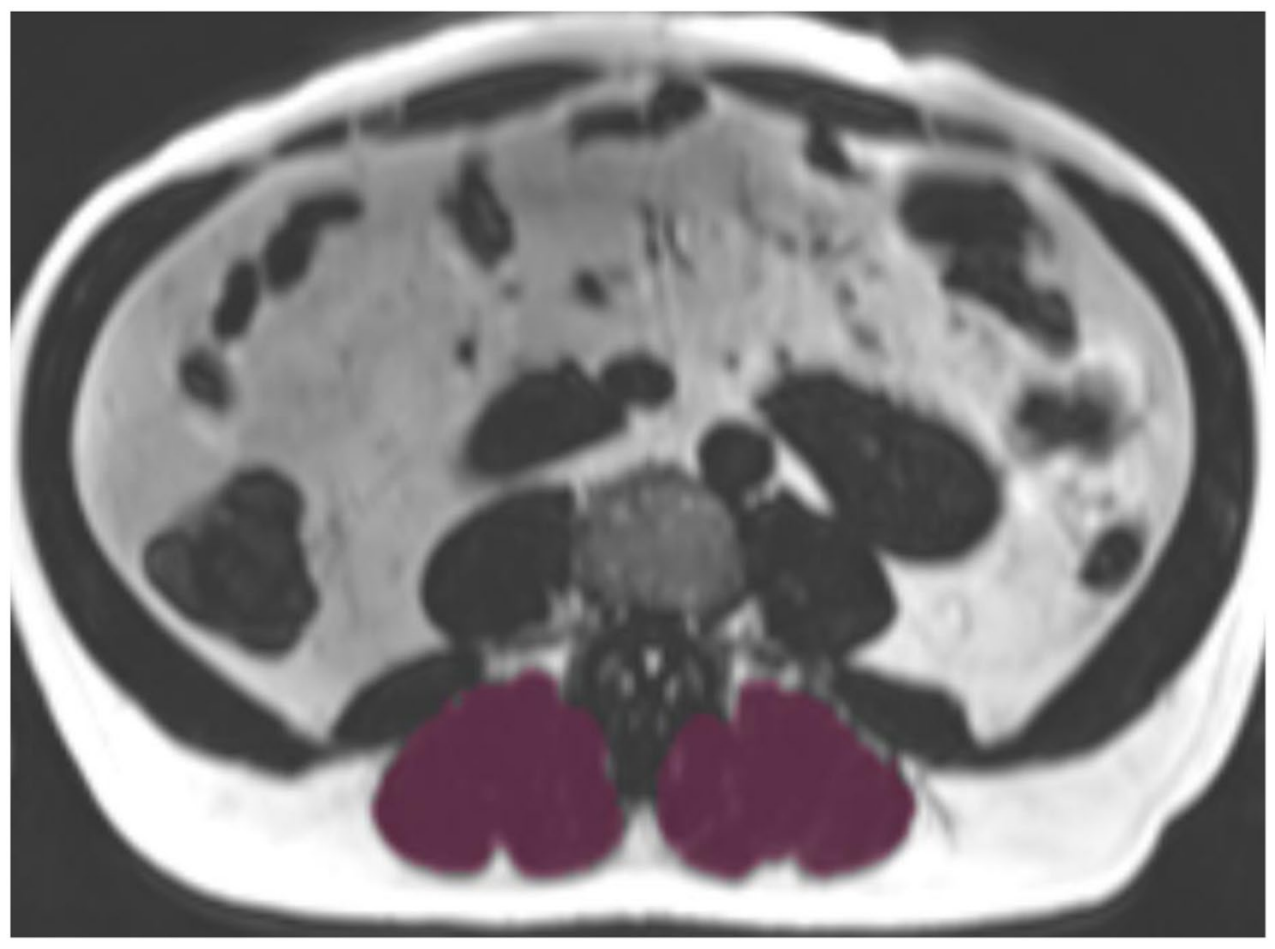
Representative axial T2*-corrected multi-echo Dixon MRI slice (fat contrast) at level L3 with superimposed paraspinal muscle segmentation (purple). Manual segmentation was performed bilaterally using standardized anatomical landmarks Abbreviations: MRI: magnetic resonance imaging; L3: 3rd lumbar vertebra
